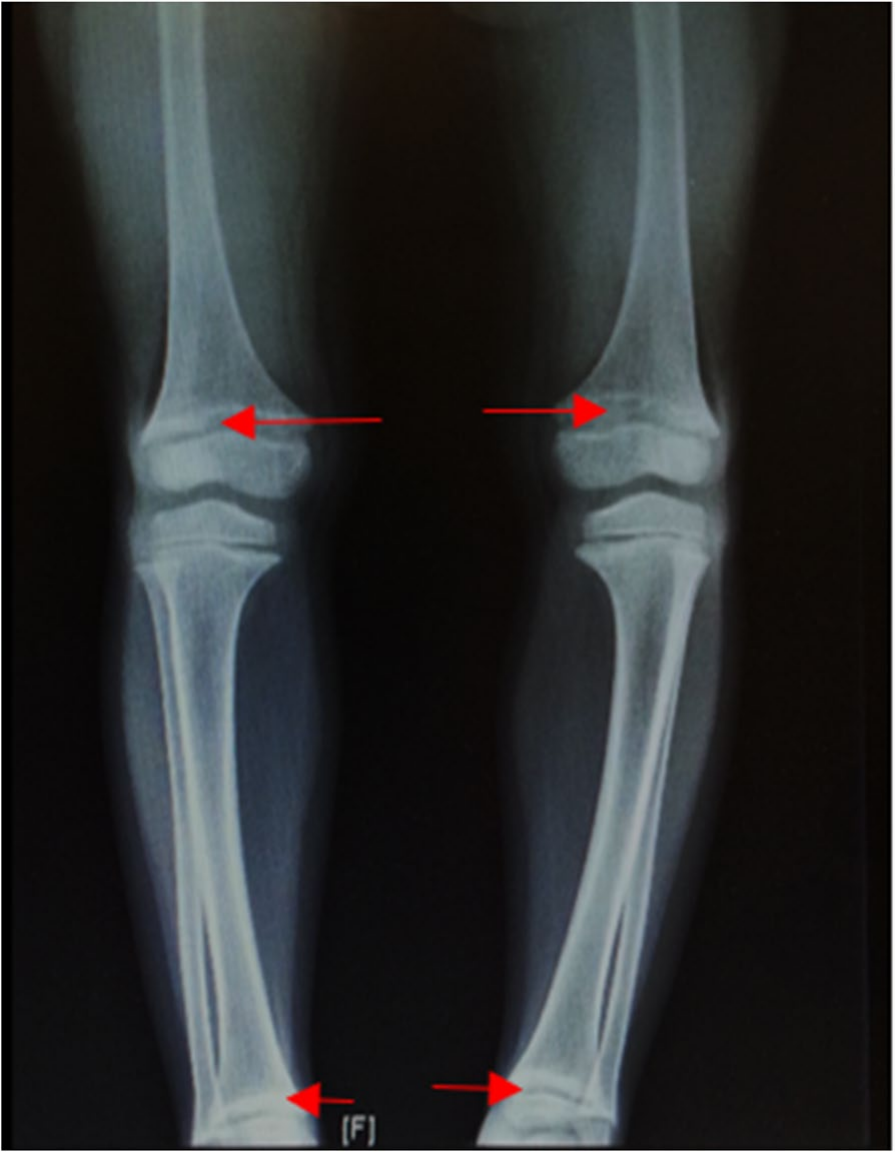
Radiograph of lower limbs of patient 1. Radiograph shows features of rickets with fraying and splaying of the metaphyses of the distal femur and distal tibia (red arrows)
